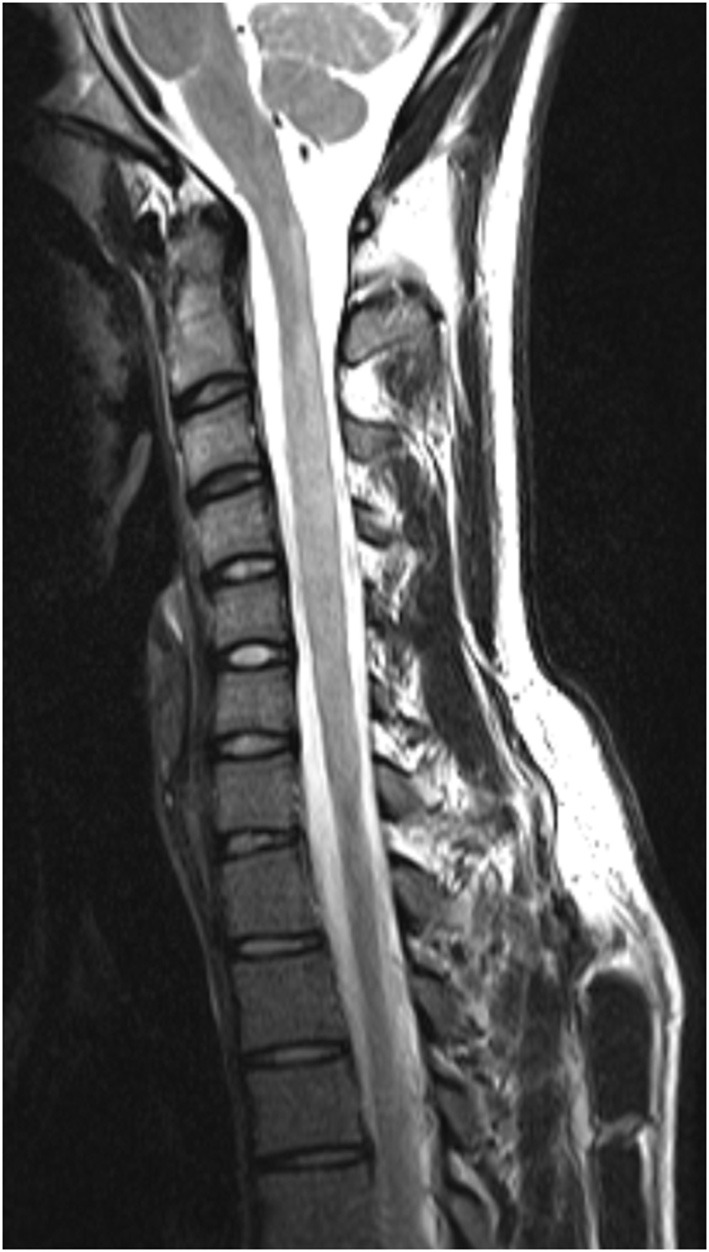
“Pseudo-longitudinally” extensive spinal cord lesion seen in multiple sclerosis. Sagittal T2 MRI of the cervical spine in a multiple sclerosis control showing longitudinally extensive high signal changes extending from C1 to C6. This lesion is confluent over at least 3 vertebral segments. Prior imaging confirmed that this lesion arose as a confluence of smaller lesions over time.
